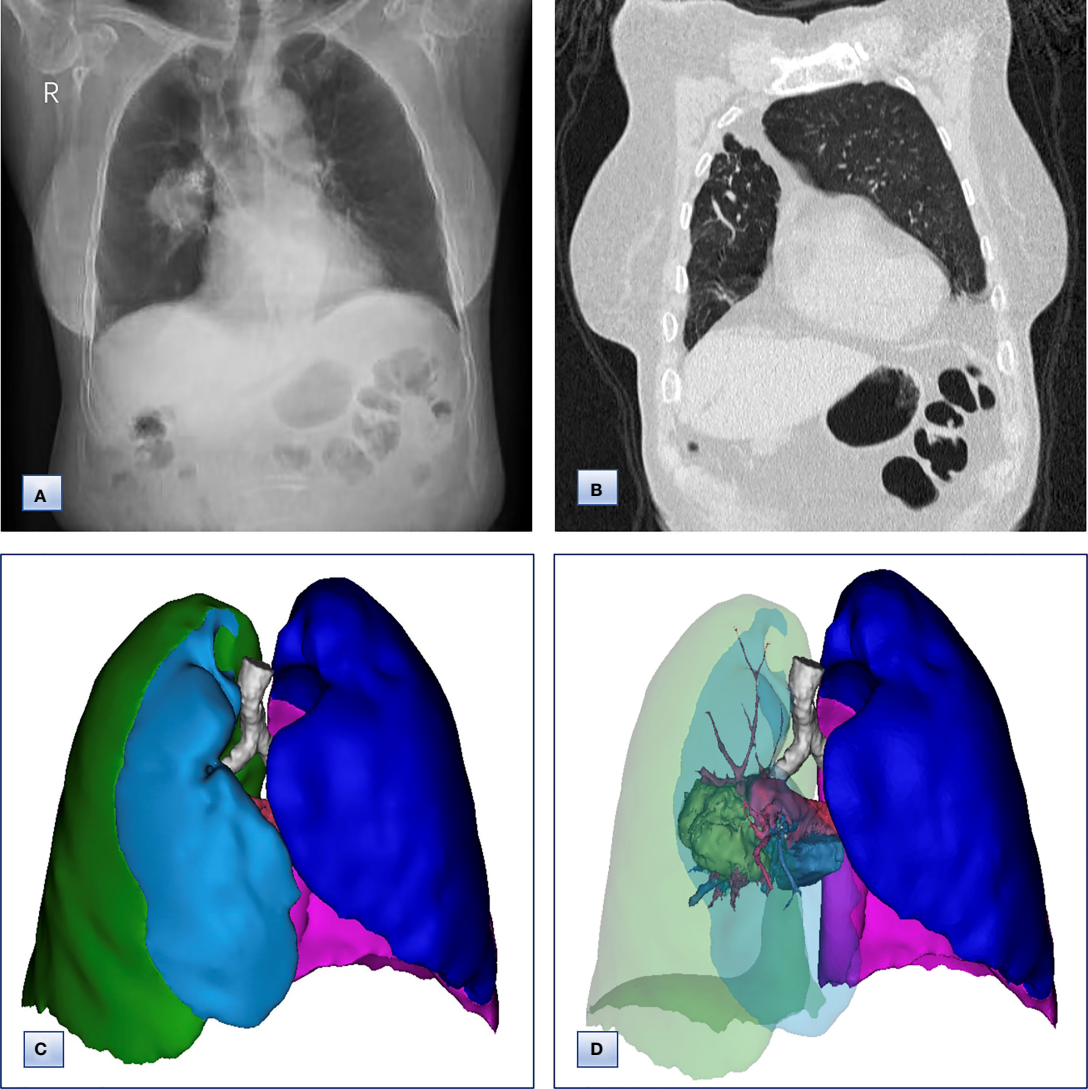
Chest radiographs, computed tomography and 3D CT reconstruction before surgery. (A) Chest radiographs, revealing that the trachea and mediastinum were shifted to the right. (B) Coronal chest CT images, indicating the absence of a horizontal fissure anterior to the aorta, with anterior herniation of the left lung crossing the midline. (C, D) 3D reconstruction of CT images, showing (C) only two lobes of the right lung and (D) complete absence of lung tissue from the right upper lobe, including the lung parenchyma, pulmonary vessels and bronchial tree.
